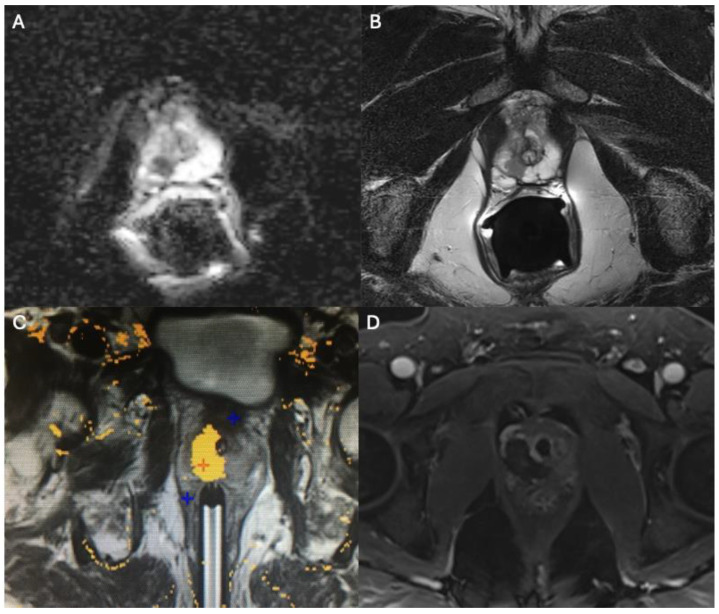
MR-guided focal laser ablation (MR FLA) of a right apical Gleason 3 + 4 prostate cancer lesion. (A,B) Pre-procedural multiparametric MRI shows the target lesion as hypointense on axial T2-weighted imaging (A) with corresponding restricted diffusion on the ADC map (B). (C) Intraoperative real-time MR thermometry. The yellow overlay indicates the estimated thermal damage zone (>60 °C), demonstrating a highly conformal ablation that completely spares the adjacent urethra. (D) Immediate post-ablation axial T1-weighted contrast-enhanced MRI. The non-enhancing region confirms successful ablation of the target lesion and preservation of periurethral tissue.
